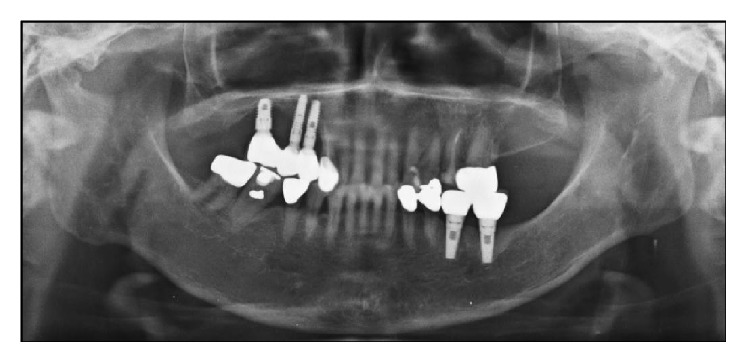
Preoperative radiographic view (panoramic X-ray image). Implants of right maxilla area show a severe alveolar bone loss lesion.
